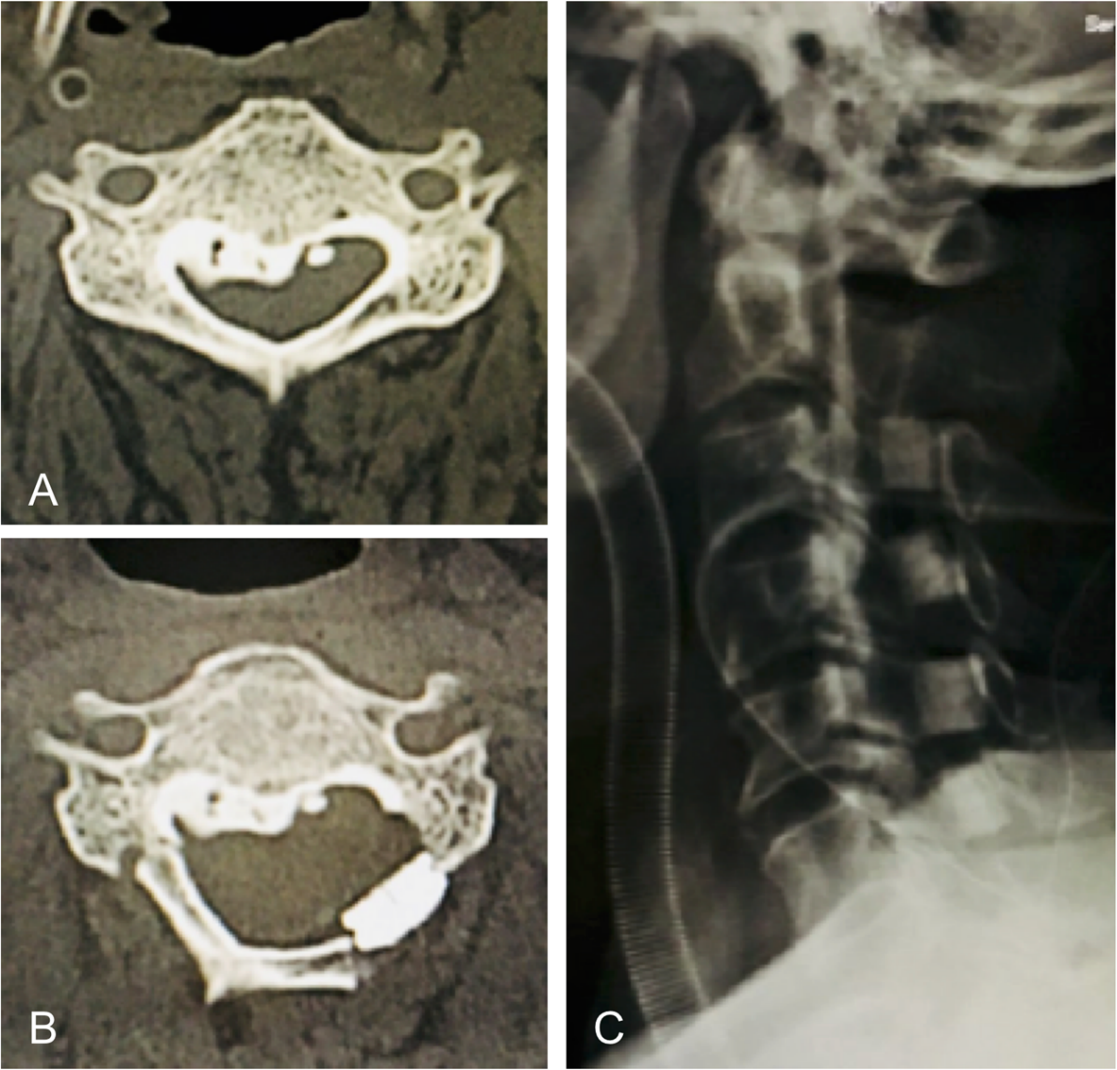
Example of a case of open door laminoplasty using hydroxyapatite block spacers. a Preoperative computed tomography image. b Postoperative computed tomography image. c Postoperative radiograph
